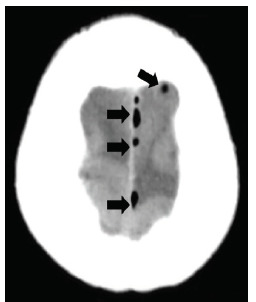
Transverse section of a computed tomography brain where foci of air (black arrows) can be seen along the left aspect of the falx cerebri outside of the superior sagittal sinus.
